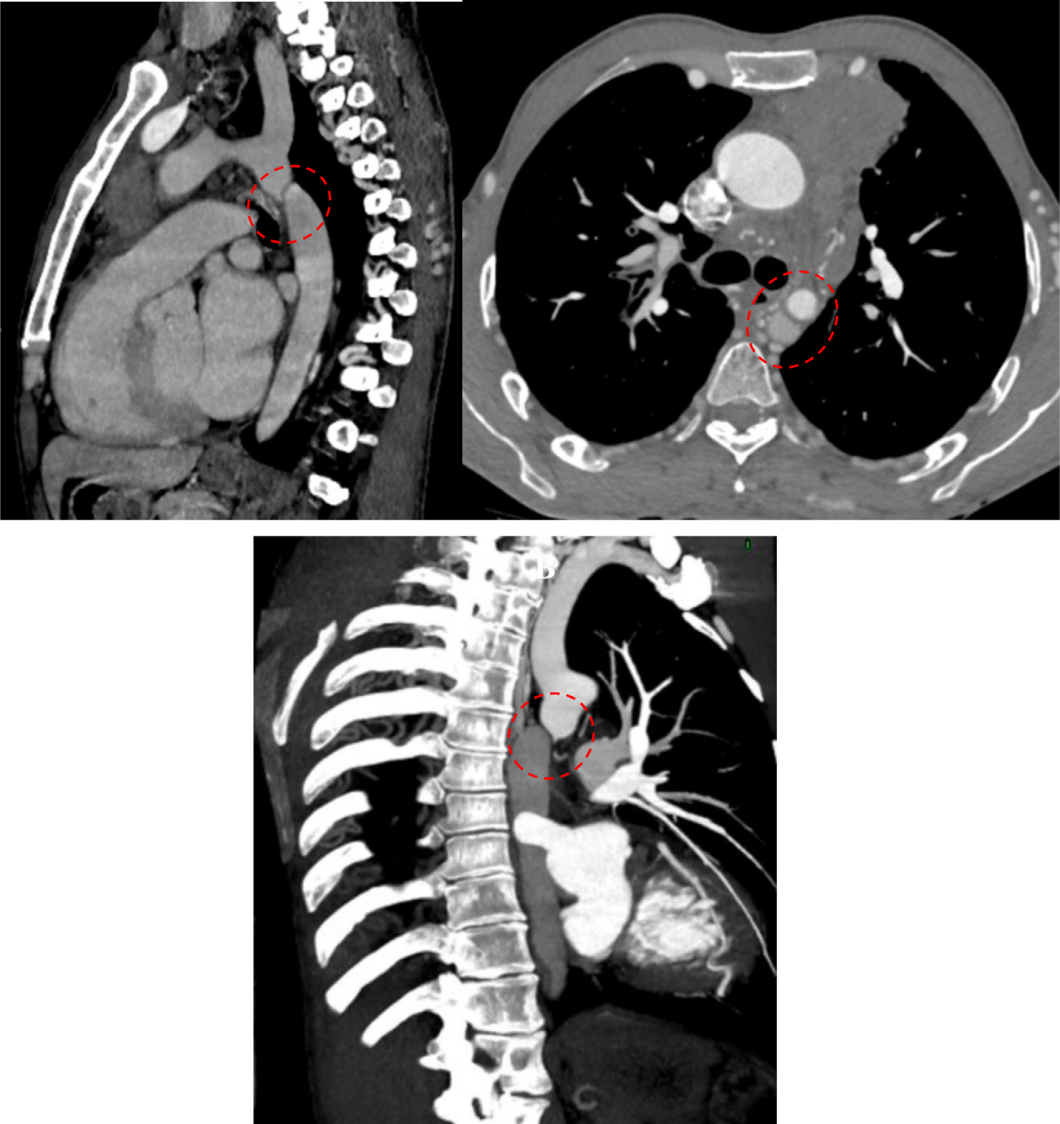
Chest CT angiography with sagittal and axial reconstructions. Critical (red circle) focal stenosis of the thoracic aorta of isthmic location, related to coarctation of the aorta, the site of coarctation is 47 mm from the left subclavian artery.
